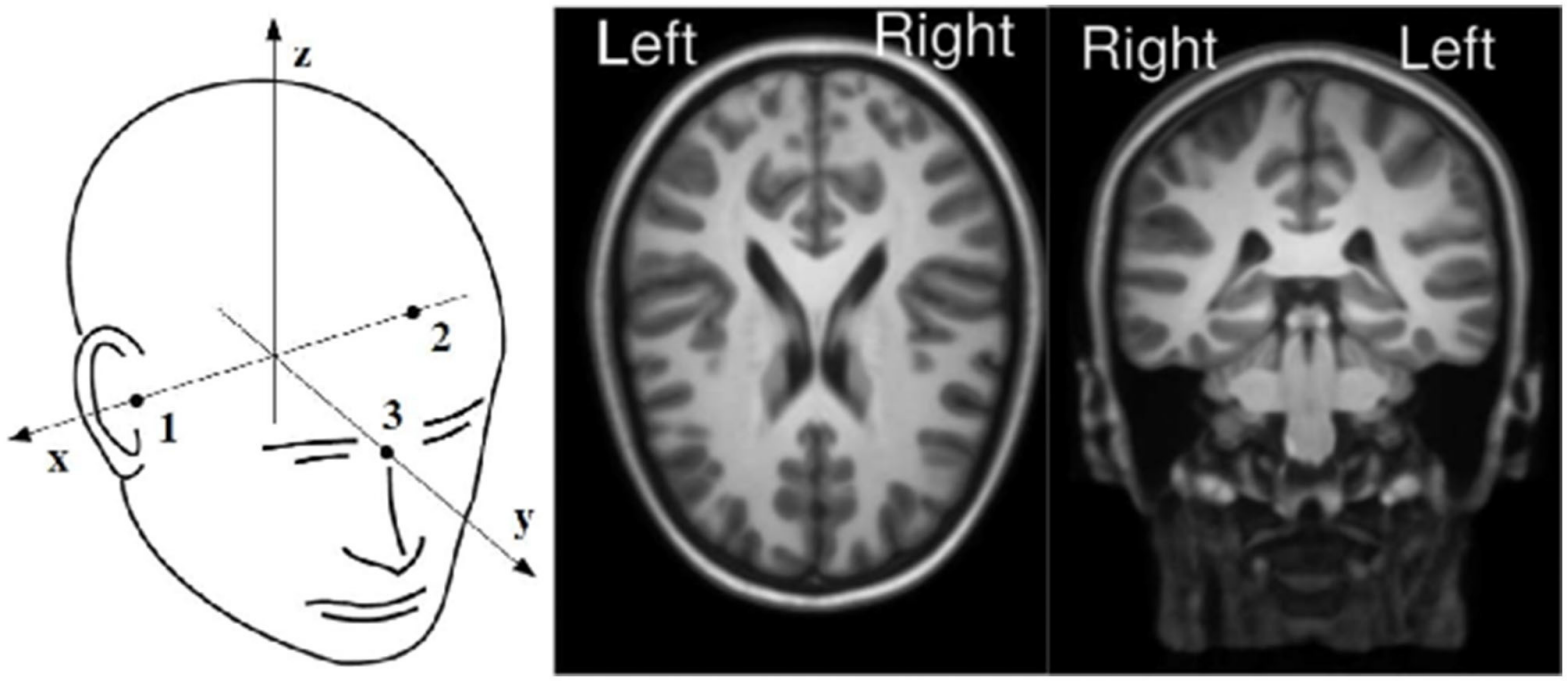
On left panel: Neuromag coordinate system. On right panel: MRI orientations used for the visualization of the dipoles fitted.
